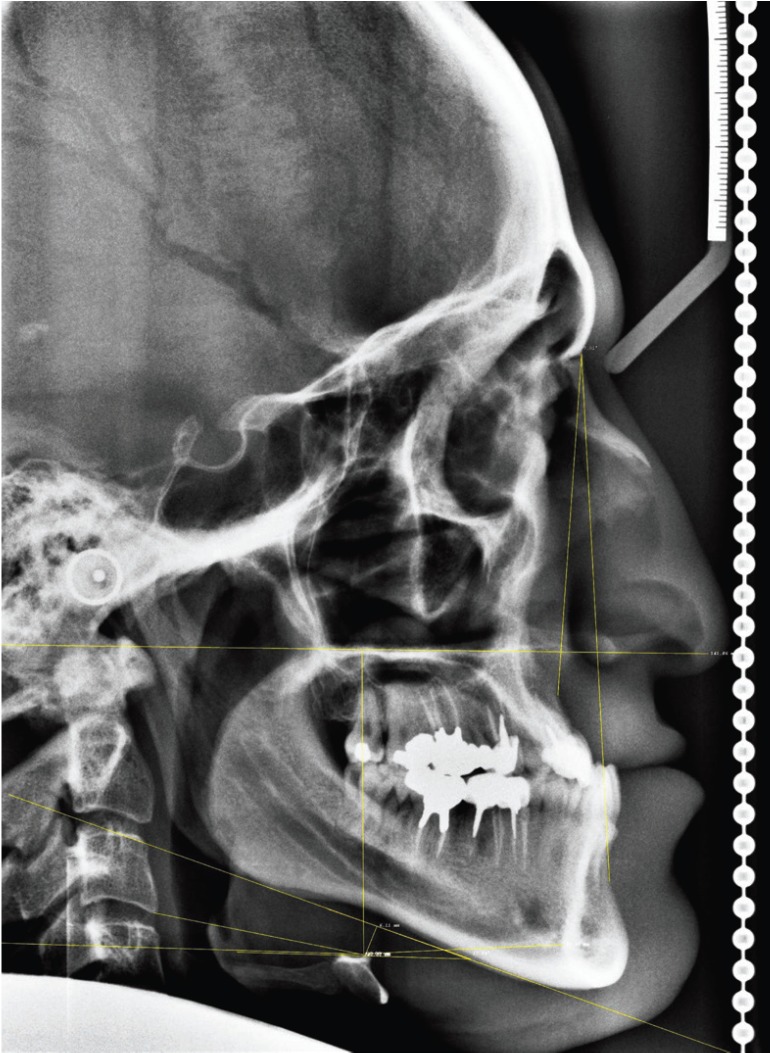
Linear and angular measurements of hyoid bone position on the lateral cephalometric radiograph used in the study.
